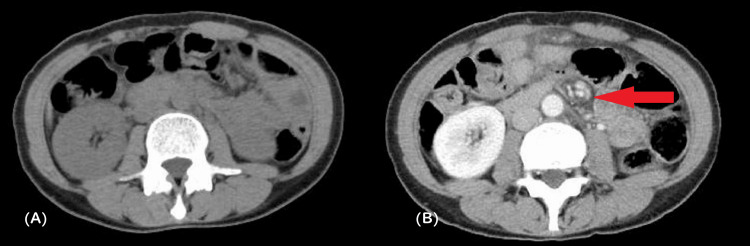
CT abdomen. 1A: Postoperative CT obtained 1 year after the initial gastrectomy showed no evidence of a whirl sign.
